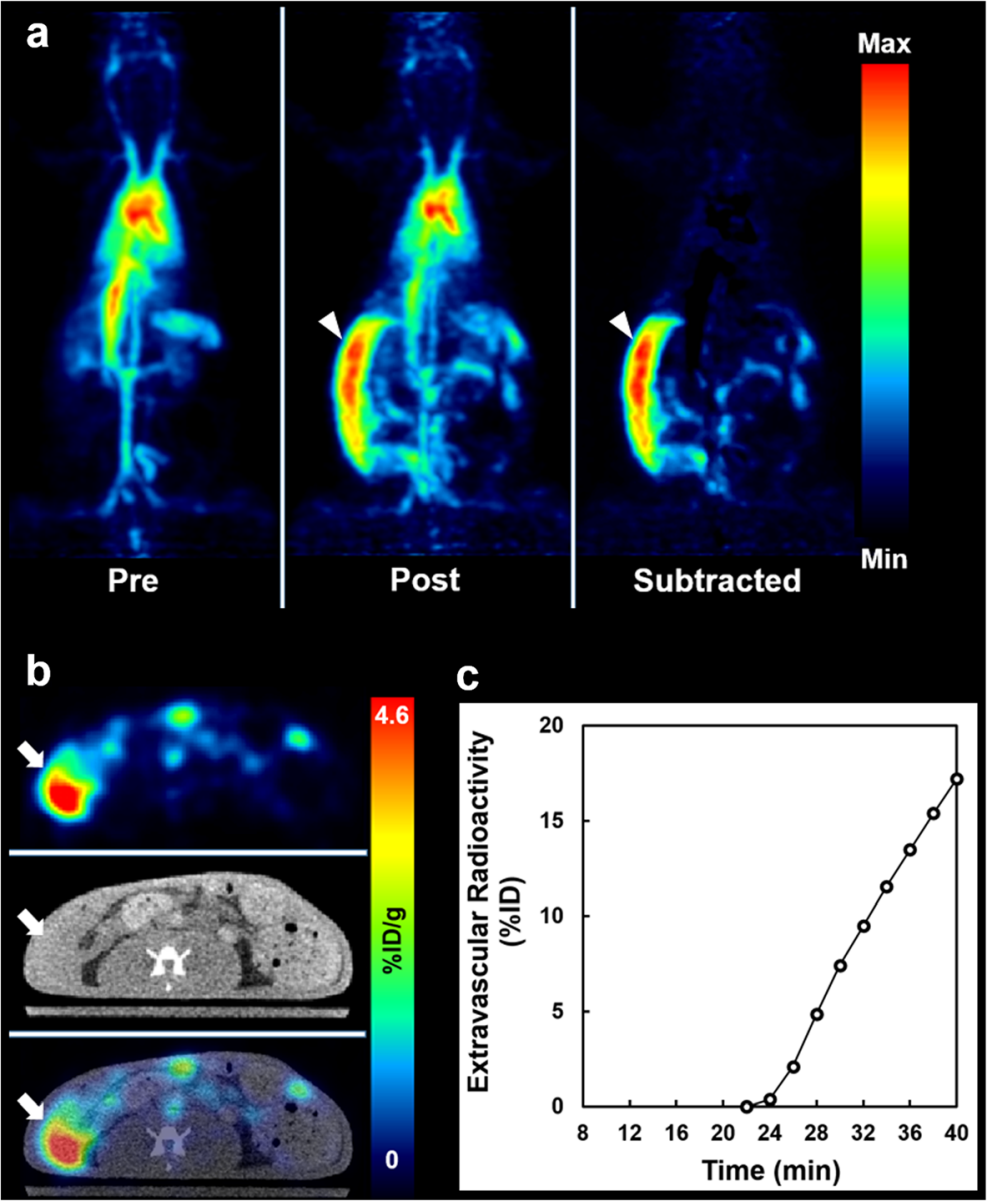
^18^F-FDG-labeled RBC PET in a persistent intraabdominal bleeding model. a Maximum-intensity-projection images in pre- (*left*) and post- (*middle*) bleeding phase and the subtracted (*right*) image between them. The subtracted image shows massive bleeding in the right side of the abdomen (*arrowheads*). b PET, CT, and fused PET/CT axial images at the middle level of the abdomen. CT shows an area of fluid collection, and the fused image shows high radioactivity in the corresponding area (*arrows*). c Time-activity curve of extravascular radioactivity in the subtracted image. The radioactivity was continuously increased after the manual puncture of the colonic wall (22 min)
